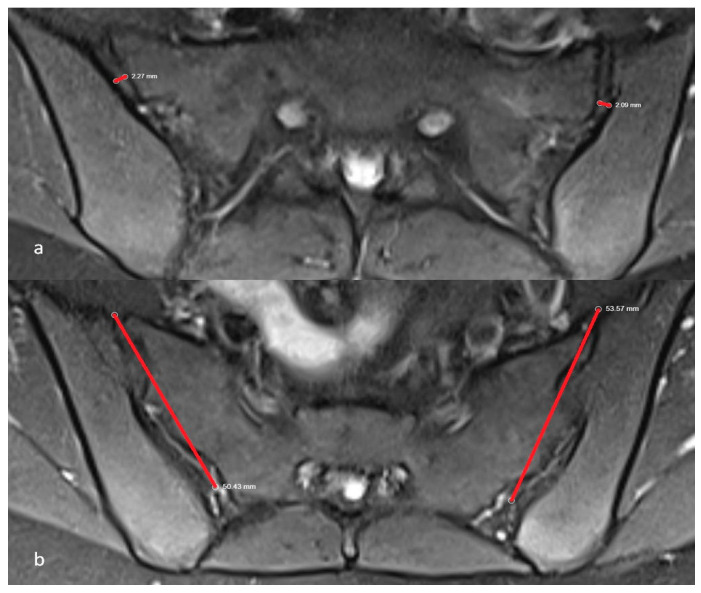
Axial proton density fat-suppressed MRI images at the level of the S2 vertebra: (a) The SIJ space; (b) The anteroposterior lengths of the SIJ.
